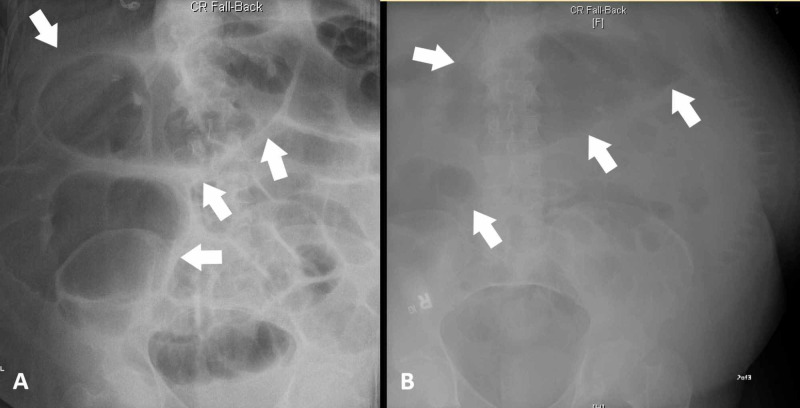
(A) Abdominal X-ray in the supine view acquired before the colonoscopy revealing multiple dilated bowel loops that are seen throughout the abdomen. (B) Abdominal X-ray acquired after the decompressive colonoscopy showing some air-filled loops less pronounced than before.
